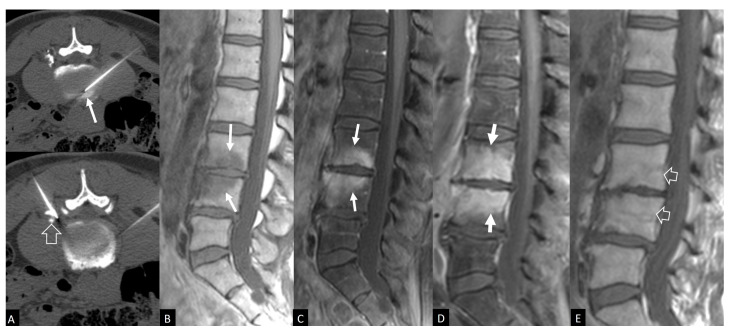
Spondylodiscitis in a 57-year-old female, 3w after CT-guided treatment with radiopaque gelified ethanol injection in the disc. (A) Axial CT images with the patient prone, show the needle placement within the L3-L4 disc and the injected material (arrow) and the needle placement close to the L3 exiting root-perineural injection with contrast (open arrow). Sagittal T1-w (B) and fat suppressed contrast-enhanced T1-w (C) MR images 3w after the procedure, show bone marrow edema (arrows). (D) Sagittal fat suppressed contrast-enhanced T1-w (D) MR image 5w after the procedure, shows deterioration of the bone marrow edema (arrows). (E) Sagittal T1-w MR image 8 months later, shows restoration of the normal bone marrow signal (open arrows) in keeping with complete resolution of symptoms. (Case courtesy: K. Spanakis).
